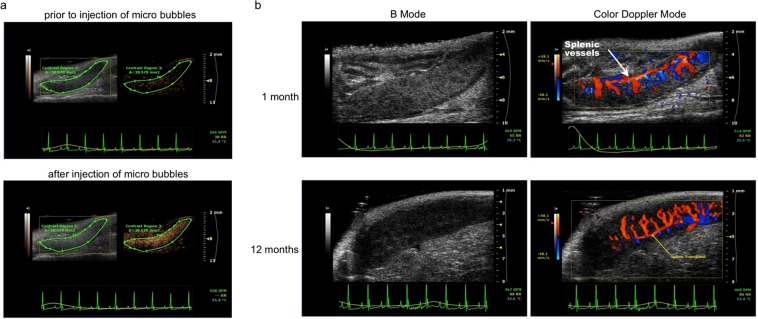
Spleen transplantation with venous and arterial anastomoses results in long term survival of the transplanted organ. Visualization of spleen perfusion with contrast ultrasound 1 week after transplantation (a). Left panels show B-mode scans. Right panels show representative contrast scans. Top 2 panels show transplanted spleen prior to injection with microbubble contrast agent. Bottom 2 panels show transplanted spleen after injection with contrast microbubbles. Colored doppler ultrasound scans showing blood flow in the spleen 1 month (top panels) and 12 months (bottom panels) following transplantation (b). Oxygenated arterial blood is red. Deoxygenated venous blood is blue.
